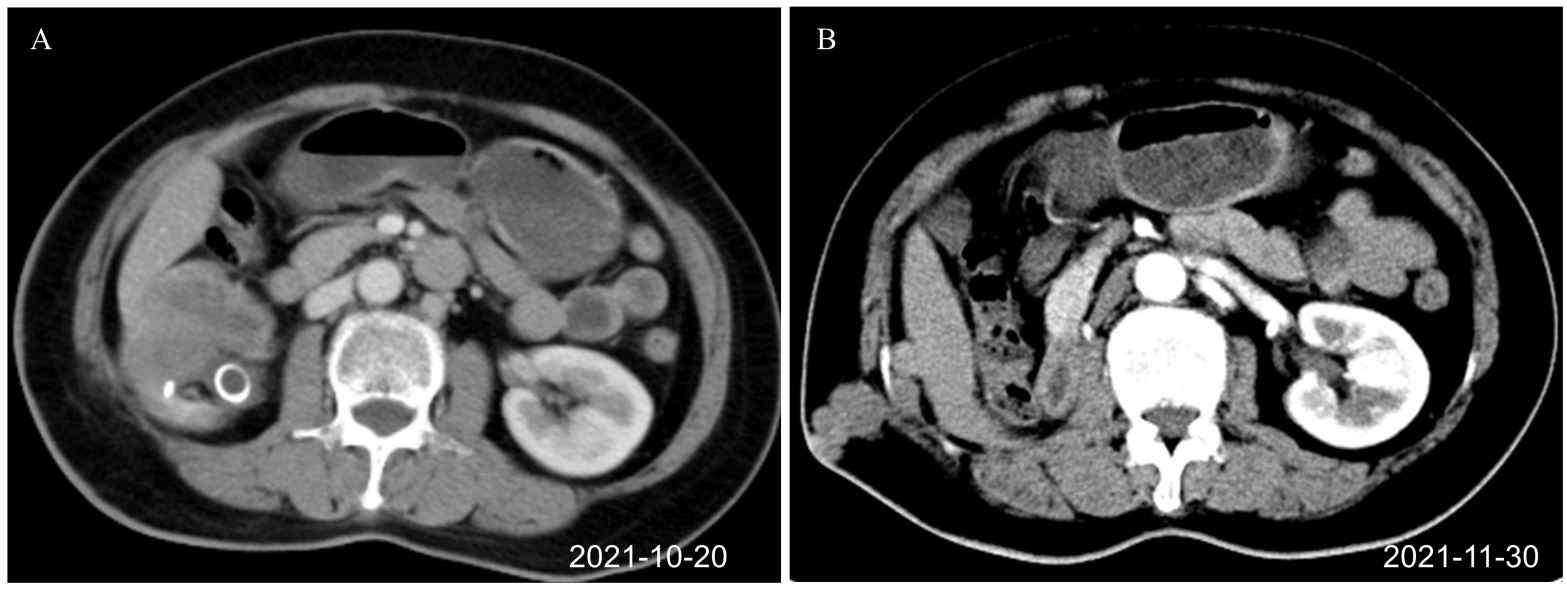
Renal CT images before combination therapy of toripalimab plus anlotinib (axial plane). (A) Right renal pelvis tumor before surgery, J-tube drainage placed in the external hospital (October 20, 2021). (B) Recurrence after the first operation. Tumors were seen at the scar of the drainage tube operation and around the liver (November 30, 2021). CT, computed tomography.
